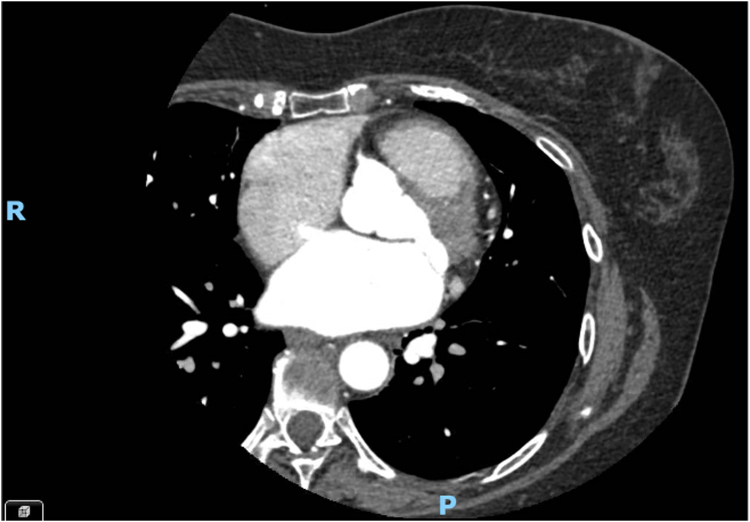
Pre-operative computed tomography scan of atrial septal defect.
